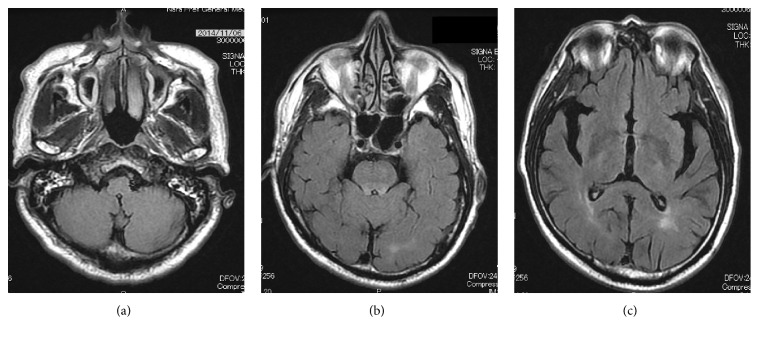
MRI (FLAIR images) of the brain after treatment. The multiple high-intensity lesions significantly improved. ((a) Cerebellar lobes lesions, (b) occipital lobes and dorsal brain stem lesions, and (c) left thalamus and occipital lobes lesions.)
